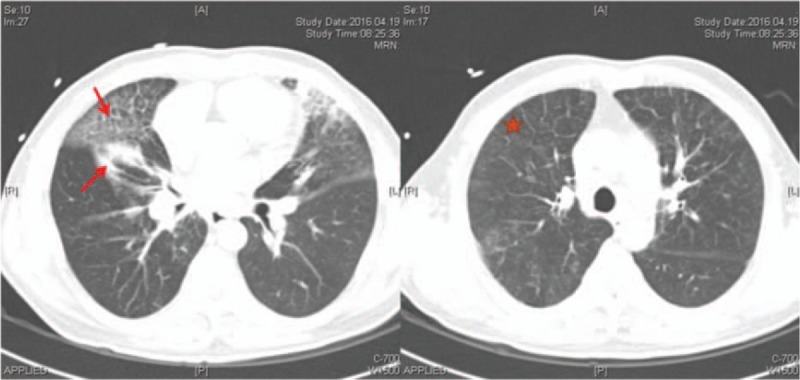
Chest CT (pulmonary window) in patient with MT. Note the findings of pulmonary consolidation (arrow) and pulmonary edema (asterisk).
